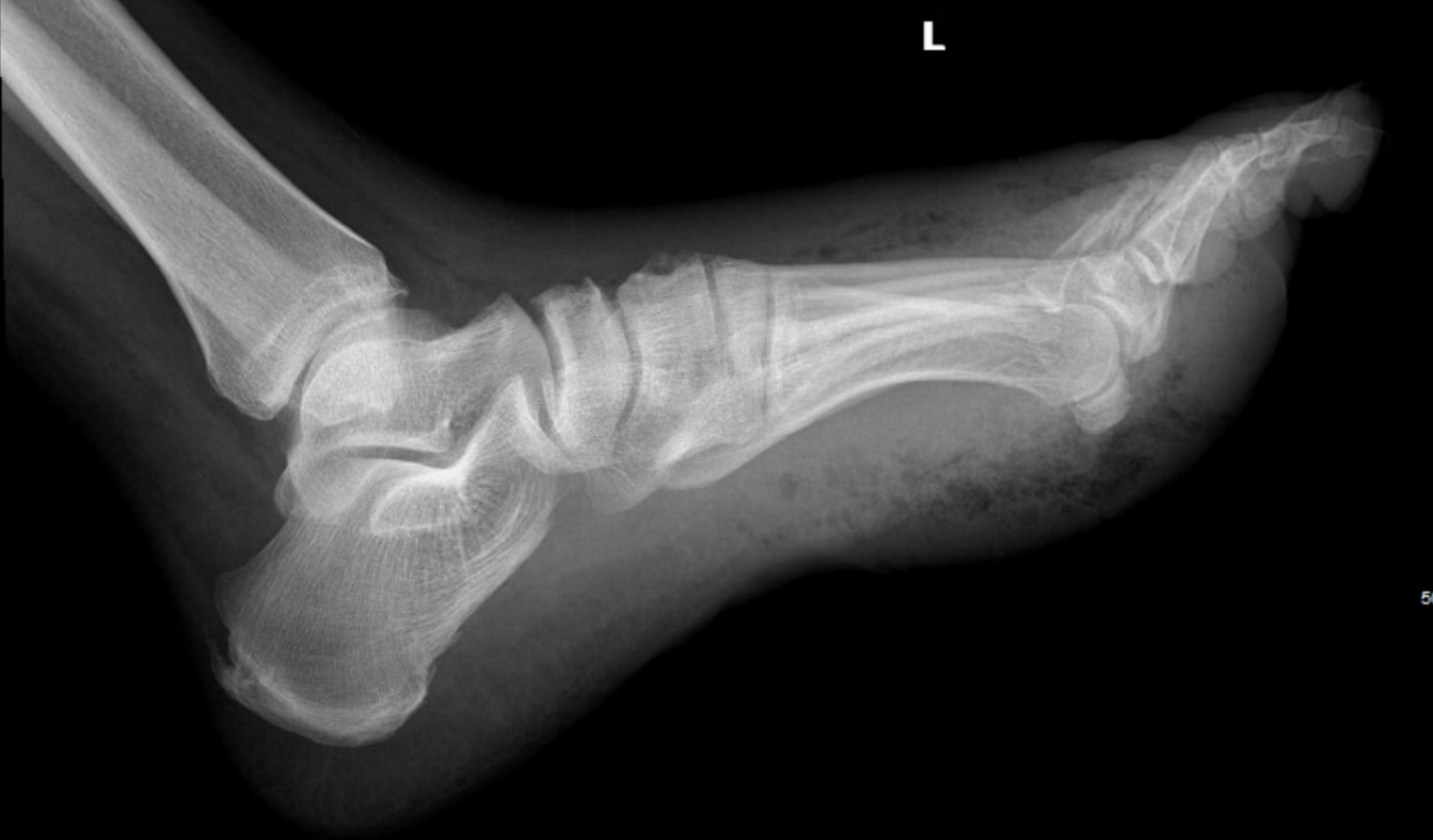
Lateral view of a plain radiograph showing extensive subcutaneous air concerning for necrotizing fasciitis.
